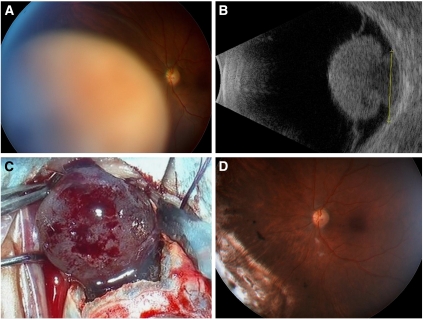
(A) A 44-year-old man was referred with an inferonasal choroidal melanoma in the left eye, which had a visual acuity of 6/7.5. (B) On ultrasonography, the tumour had a collar-stud shape and measured 15.3 mm in basal diameter, with a thickness of 11.2 mm. (C) The patient underwent transscleral local resection under hypotensive anaesthesia with adjunctive ruthenium plaque brachytherapy. (D) At 6 months postoperatively, the visual acuity was 6/9.5. The melanoma was of spindle-cell type with no chromosome 3 loss shown by multiplex ligand-dependent probe amplification. These findings indicate a minimal risk of metastatic disease and therefore a near-normal life expectancy.
